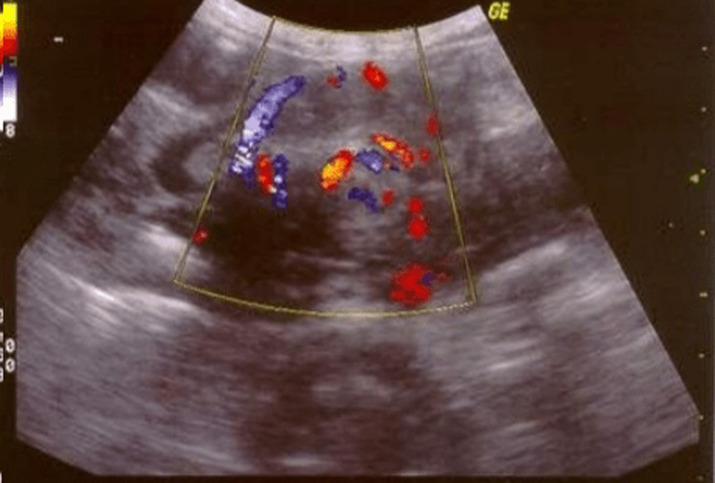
ultrasound Doppler showing malrotation vessels
